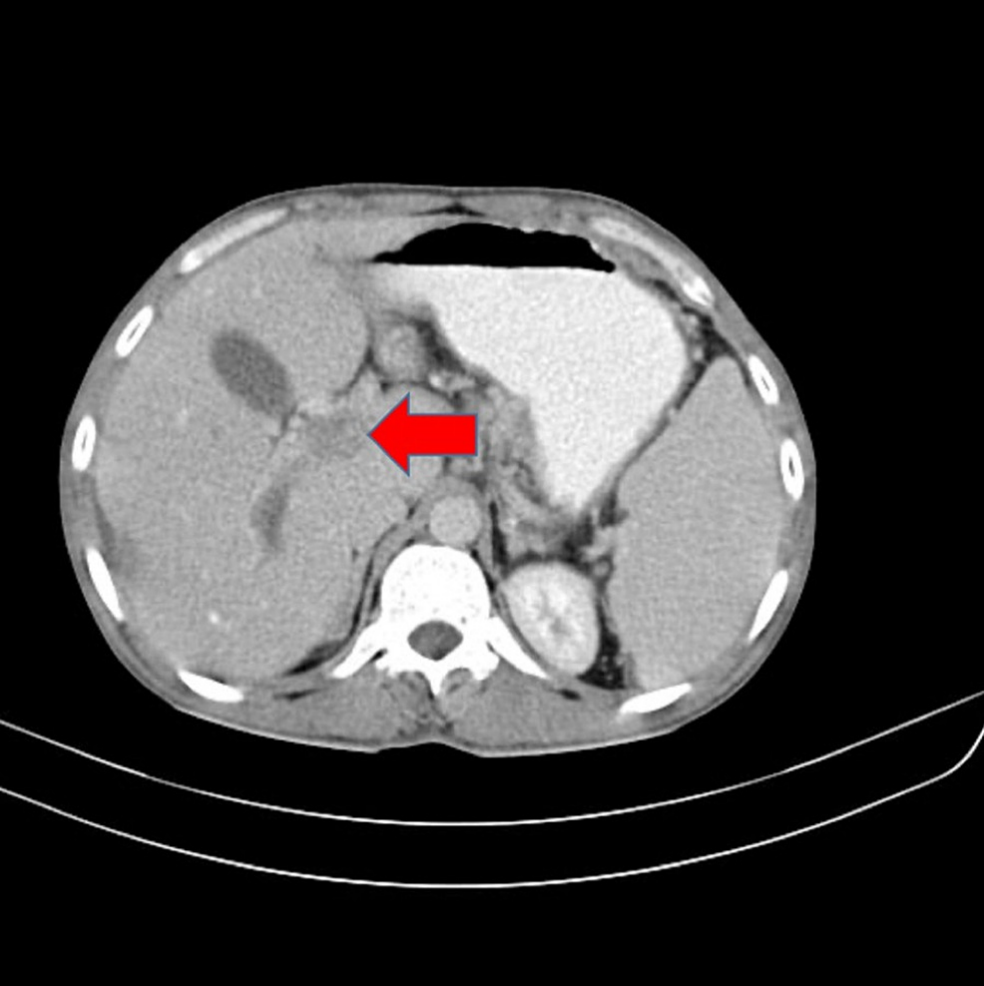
Contrast-enhanced computed tomography of the abdomen showing thrombosis of the main portal vein
